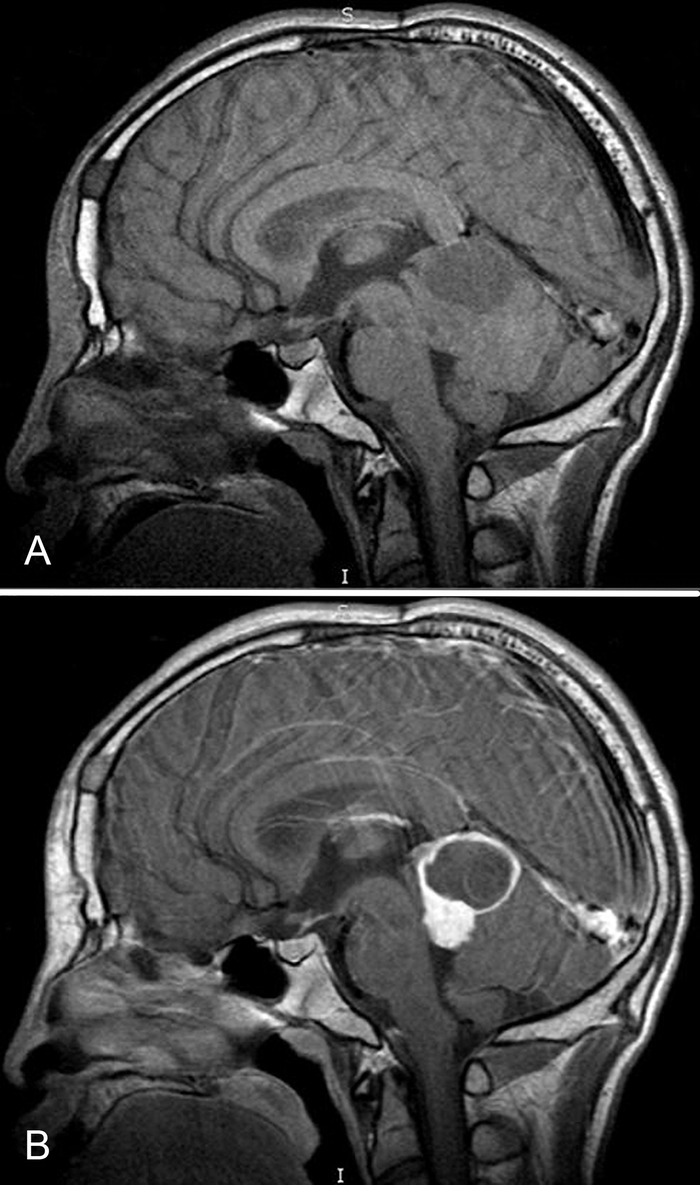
Magnetic resonance imaging without contrast (A) and with contrast (B) shows a solid and cystic enhancing mass measuring 3.9 cm × 3.6 cm × 2.4 cm centered in the region of the tectum, extending down to the superior aspect of the fourth ventricle with mass effect on the posterior midbrain and displacement of the cerebral aqueduct and with maintained patency.
